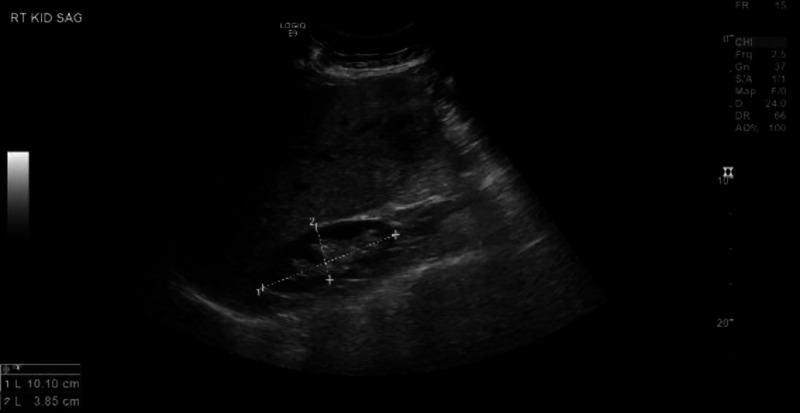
Ultrasound of gall bladder The dotted lines demonstrate the dimensions of stone inside the gall bladder
